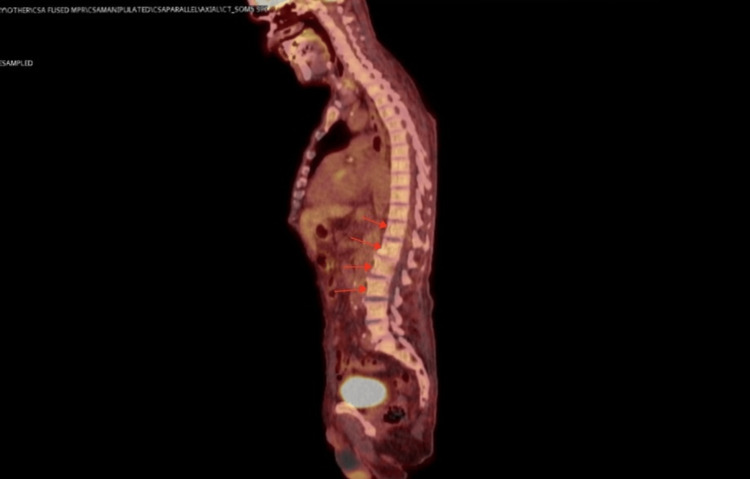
Fluoro-deoxyglucose positron emission tomography (FDG-PET) scan showing sclerotic vertebral body lesions without hyper-metabolic activity (red arrows)
